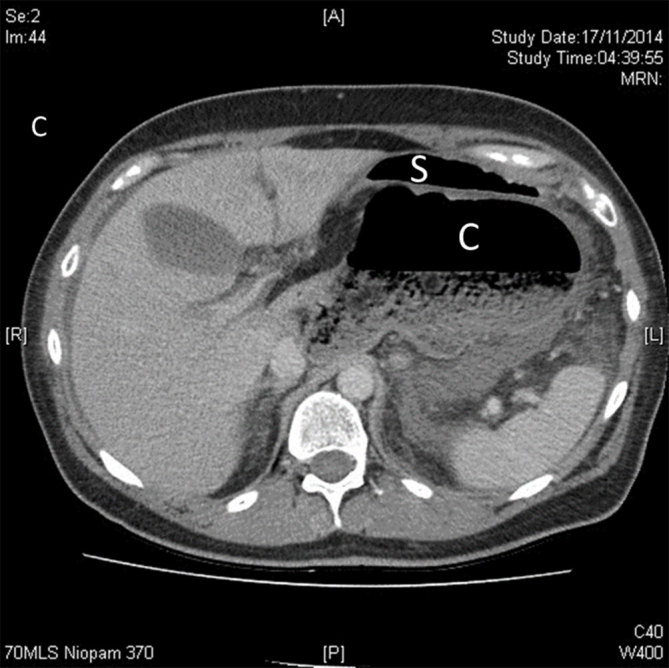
An axial section of the CT scan showing the caecum (C) behind the stomach (S).
